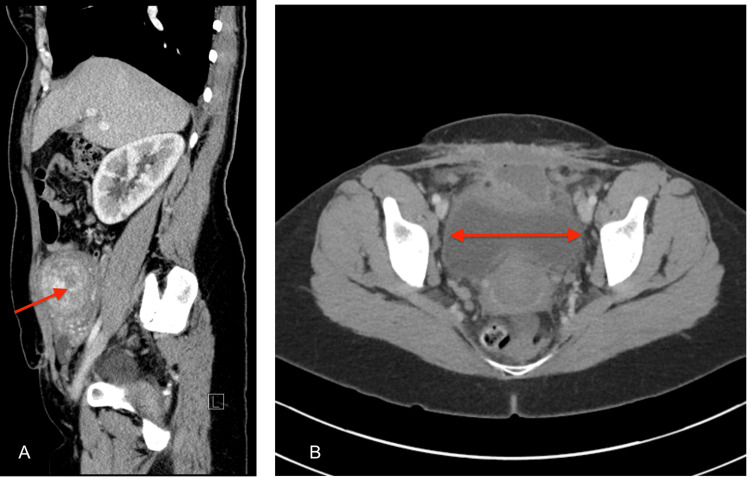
A CT scan of the abdomen and pelvis eight weeks before total abdominal hysterectomy The red arrow shows the uterine fibroid.
